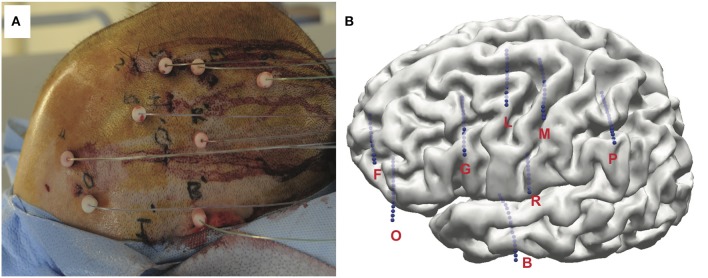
(A) Example of a final postoperative image of left frontal-temporal-parietal SEEG implantation. Subject shown did not perform task. (B) Three-dimensional MRI reconstruction showing details of subject's unique cortical anatomy and the relative position and depth of of implanted electrodes.
